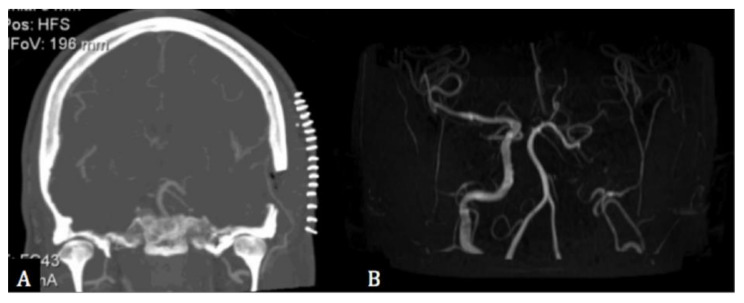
(A) Post-operative coronal computed tomographic angiography showing the bypass between the superior temporal and the middle cerebral arteries. (B) 3-years post-operative magnetic resonance angiography showing the occlusion of the left internal carotid artery and the left middle cerebral artery supplied by the bypass.
